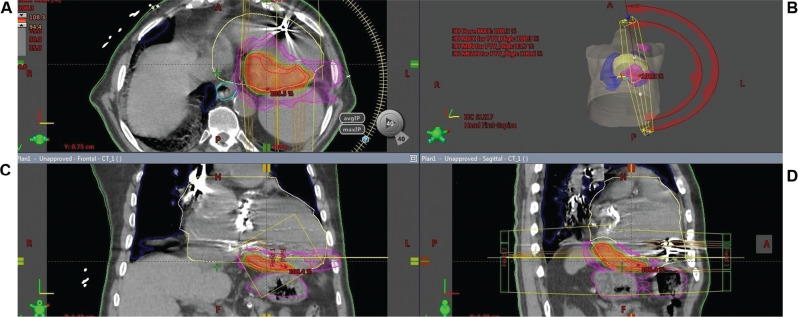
Treatment plan by the isodose distribution of SBRT in axial (A), coronal (C), and sagittal (D) orientation is shown with PTV (B). Color lines delineate areas with the same dose (isodose lines). CT, computed tomography; PTV, planned target volume; SBRT, stereotactic radioablation therapy.
